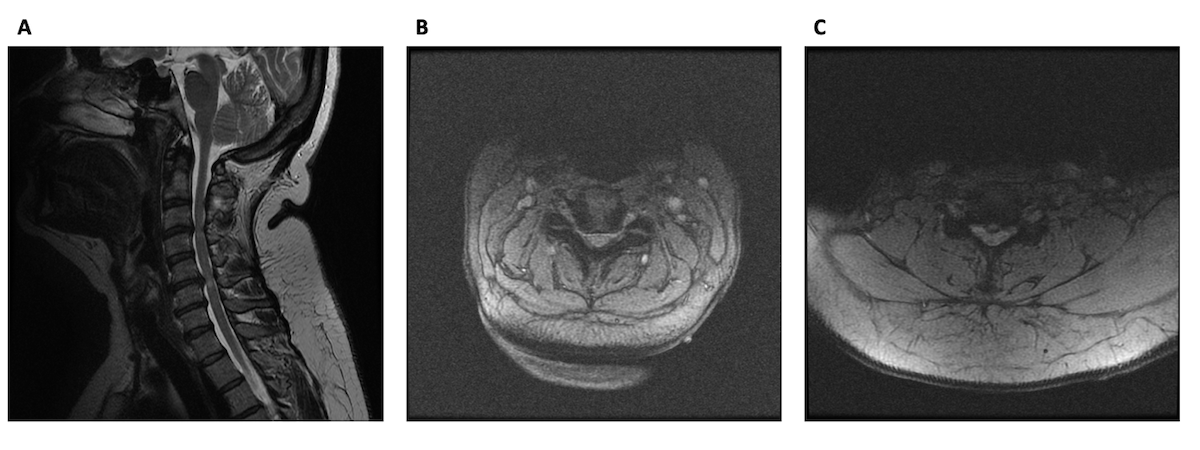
Investigations supporting a diagnosis of degenerative cervical myelopathy: (A) sagittal T2 MRI; (B) C3/4 axial T2 MRI; and (C) C5/6 axial T2 MRI. The MRI was performed 1 month before shunt revision. MRI: magnetic resonance imaging.
